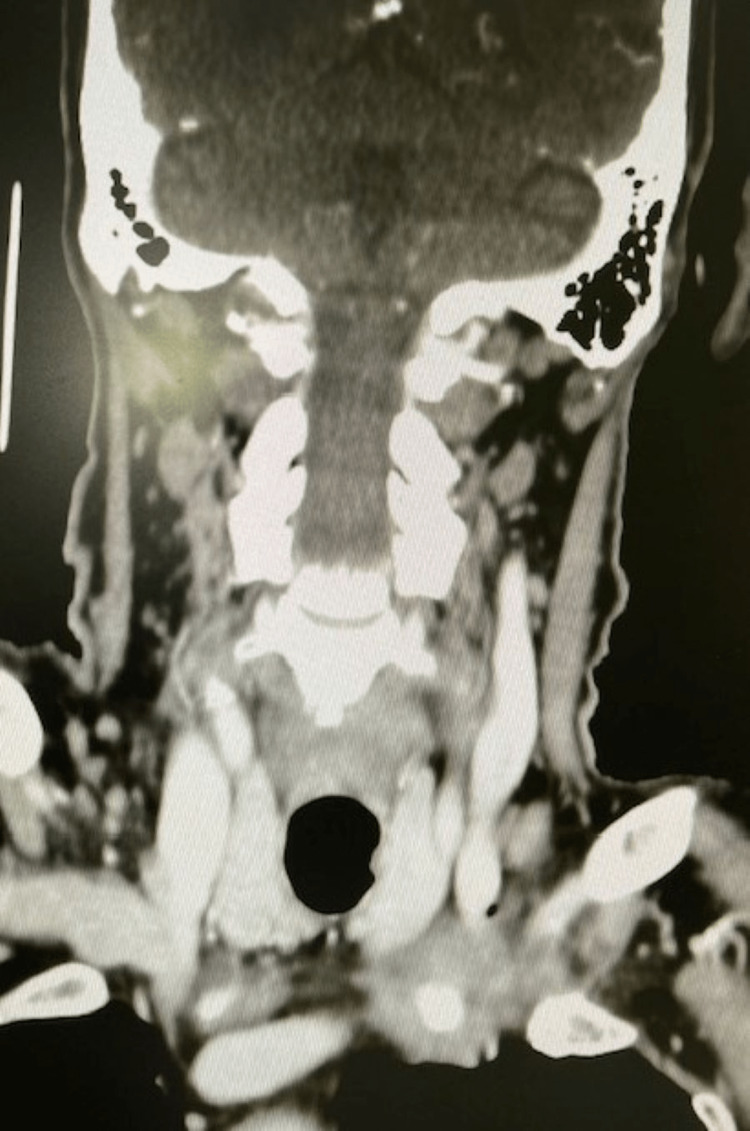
CT scan of the neck CT neck with contrast performed which detected no mass or lymphadenopathy
